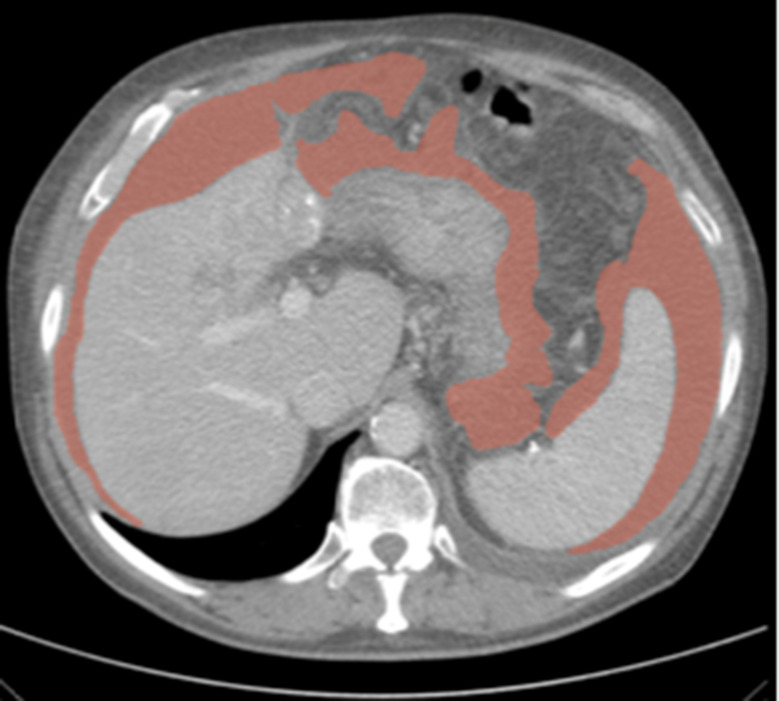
Example of AI-based segmentation (red) of ascites on axial abdominal CT
